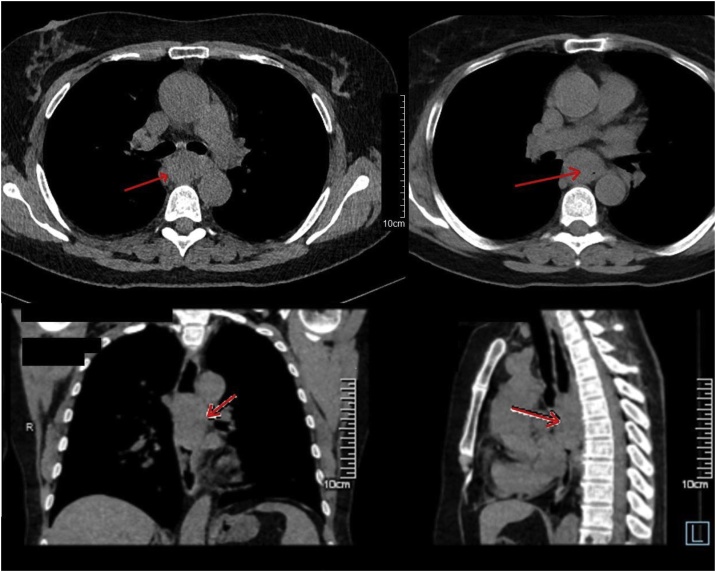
Preoperative contrast CT (see comments in the text).
